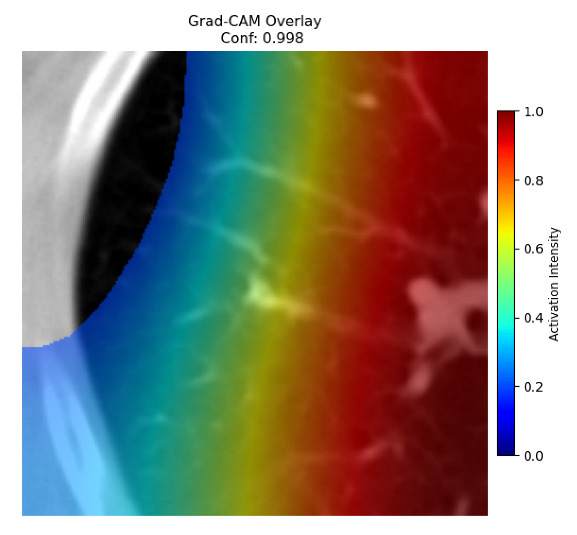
Table 7 bioengineering-13-00552-t007: LIME and Grad-CAM explanations generated by four lightweight CNN variants using ConvNeXt-Tiny as backbone, applied to four representative CT images.
